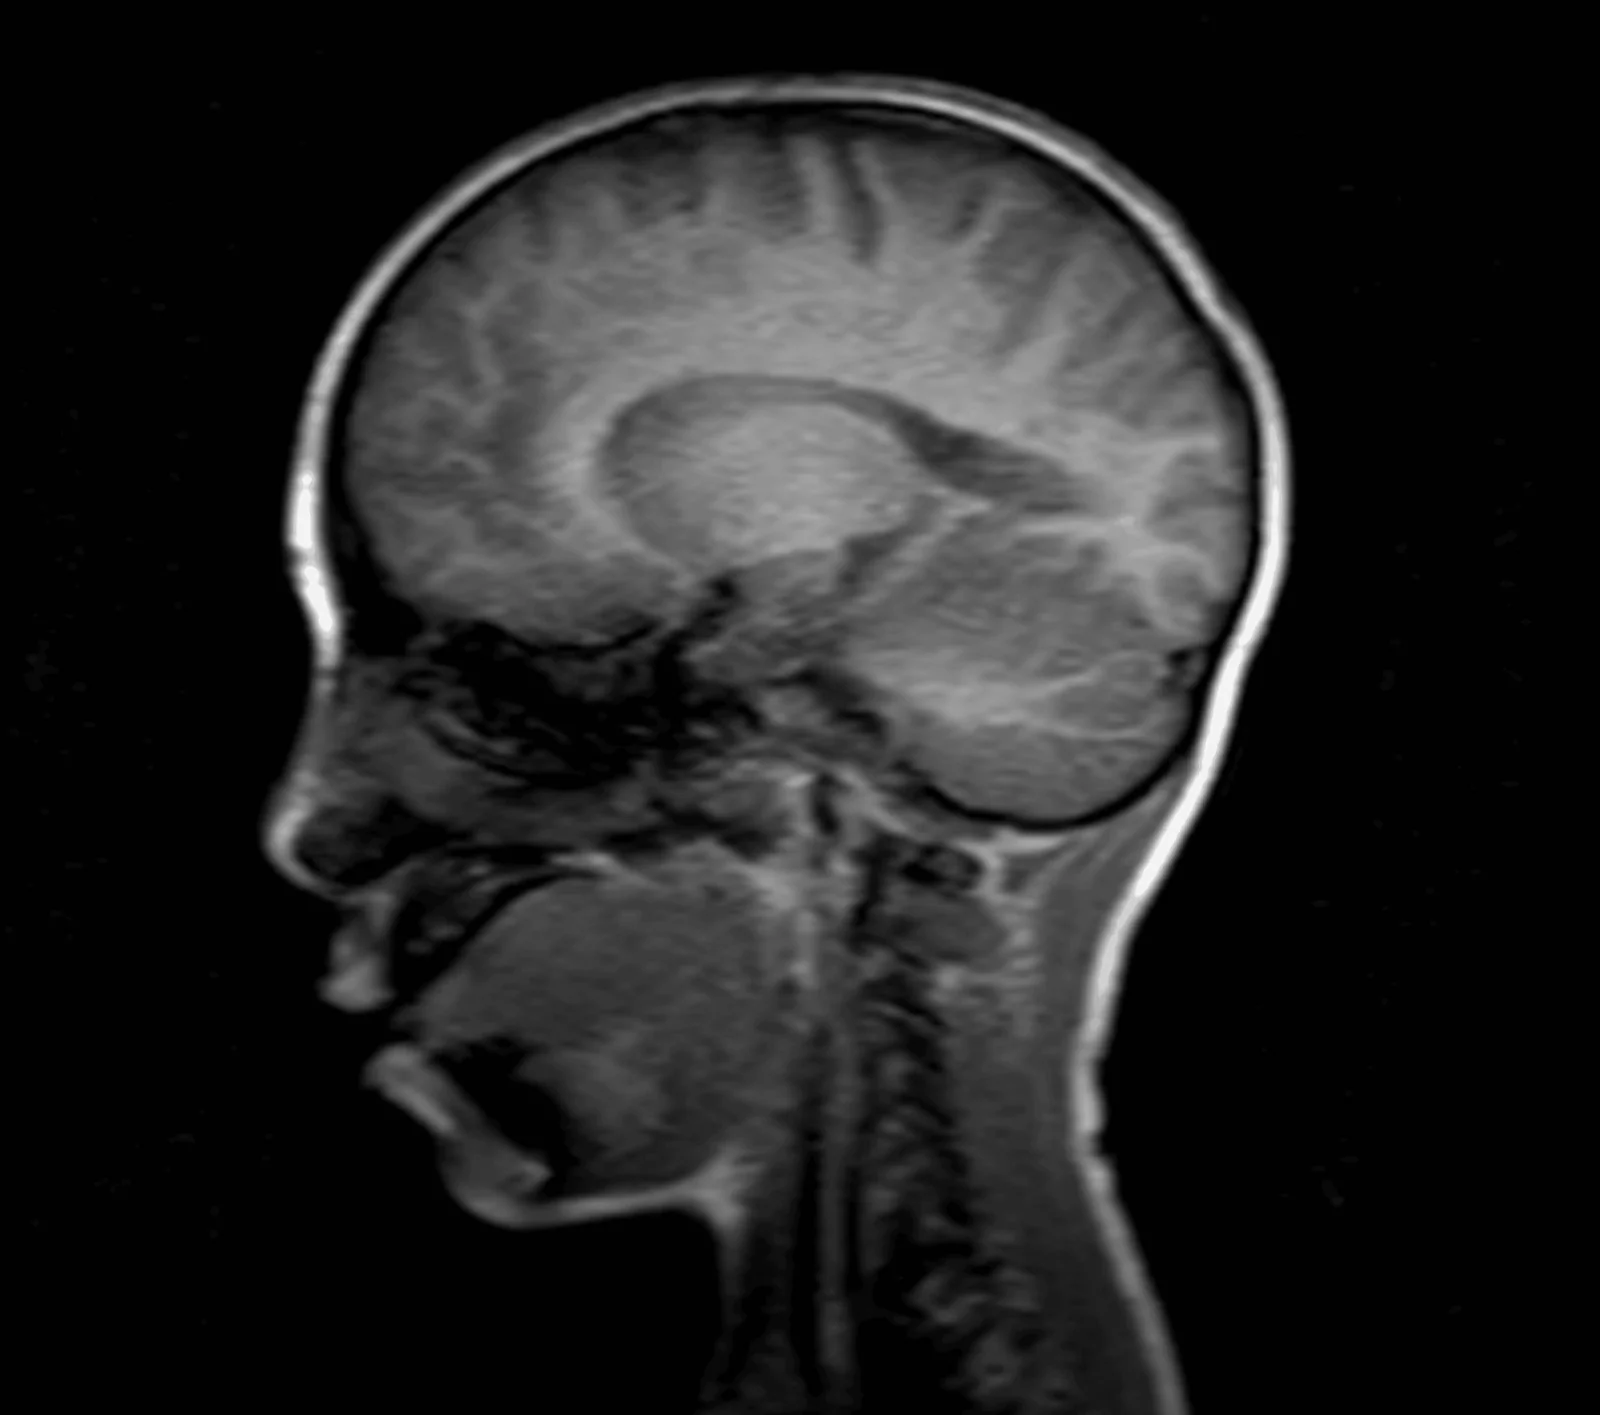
Mid-sagittal T1-weighted image. Normal cortical gyral pattern, midline structures, corpus callosum, brainstem, and cerebellar vermis. No tonsillar herniation or cervicomedullary junction abnormality.
